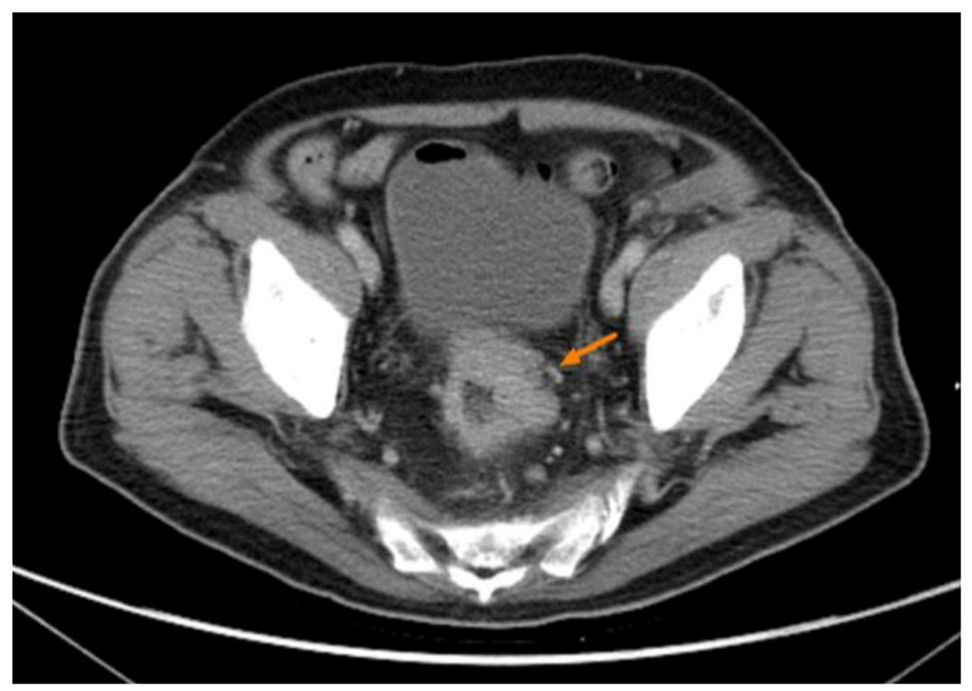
Displays the tumor found by using contrast CT
